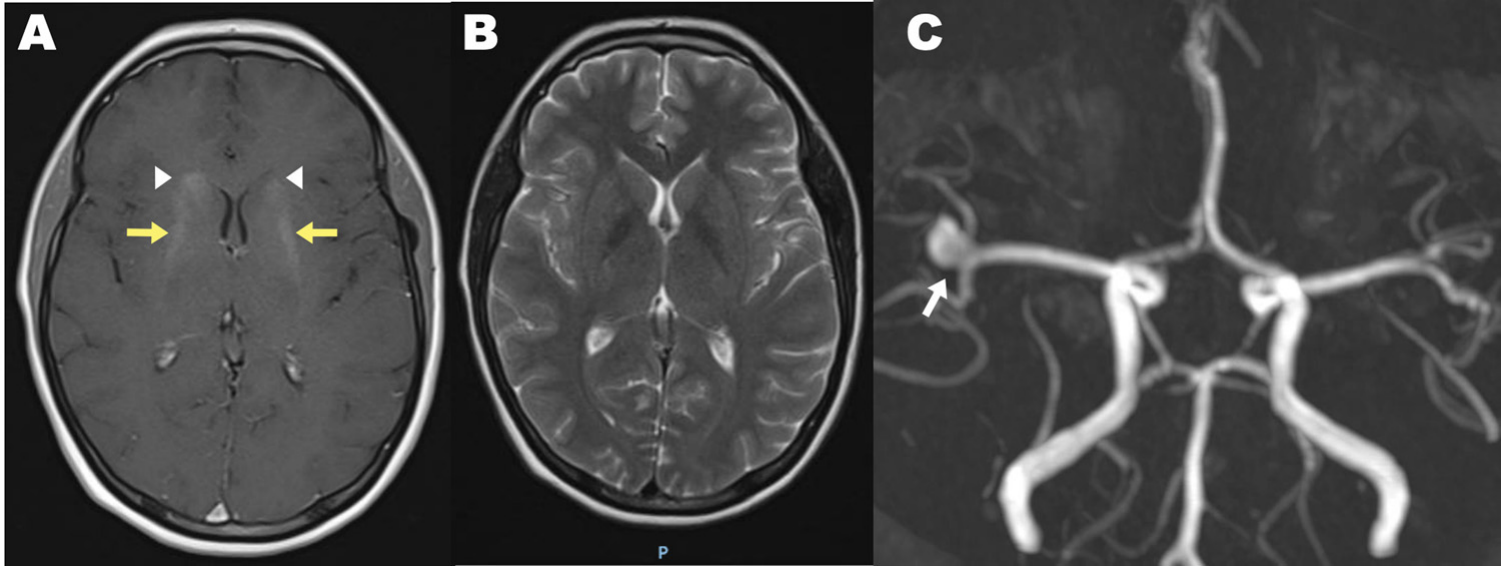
Contrast-enhanced head MRI: (A) Axial T1 weighted: bilateral symmetrical abnormal hyperintensities in the bilateral basal ganglia (white arrowheads) and corona radiata (yellow arrow). Absent in T2W images suggestive of calcifications. (B) Axial T2 weighted MRI: Absence of hyperintensities indicating lack of vasogenic or cytotoxic edema. (C) Time of Flight (TOF): Saccular aneurysm of the M2 portion of the right middle cerebral artery is observed with a longitudinal diameter of 9 mm, neck 3 mm, and dome of 7 mm.
